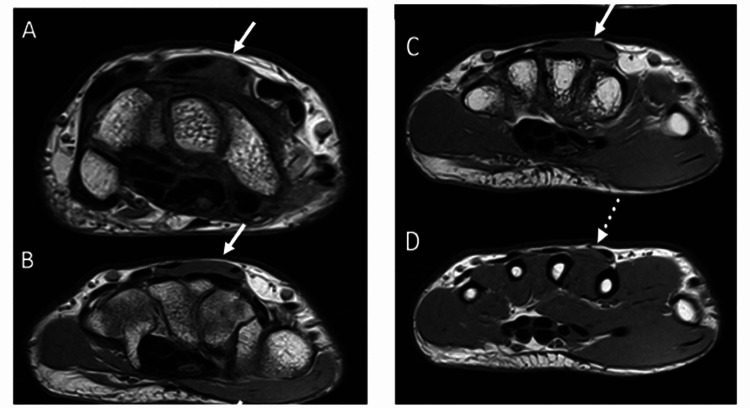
MRI hand There is an accessory muscle shown in images A, B, C, and D (white arrows) between the 2nd and 3rd extensor compartments of the dorsum of the wrist, consistent with the extensor digitorum brevis manus muscle. Triangular FibroCartilage Complex is Intact with normal morphology with no abnormal signal intensity. Scapho-Lunate and Lunotriquetral Ligament are Grossly intact. No scapholunate dissociation. Radiocarpal joint unremarkable. Distal radioulnar joint unremarkable. Midcarpal carpal joints are Grossly intact and have no erosive changes. Carpometacarpal joints are Grossly intact and have no definite erosive changes. Carpal bones are Maintained. Extensor and Flexor tendons are intact. Median Nerve is unremarkable. The ulnar nerve is unremarkable.
